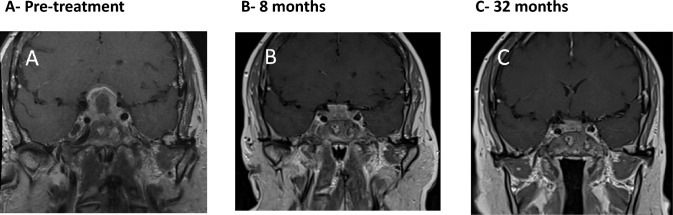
Coronal post-contrast T1W1 MR images of the pituitary. A Pre-treatment pituitary MRI scan showing a large enhancing centrally necrotic sellar and suprasellar lesion which elevates and compresses the optic chiasm. B Image 8 months following high dose GC treatment, MR images of the pituitary showing the initial response to treatment with size reduction of the sellar/suprasellar lesion. There is still minimal contact with the optic chiasm. C Pituitary MRI at 32 months, showing improvement and decrease in size of pituitary mass
